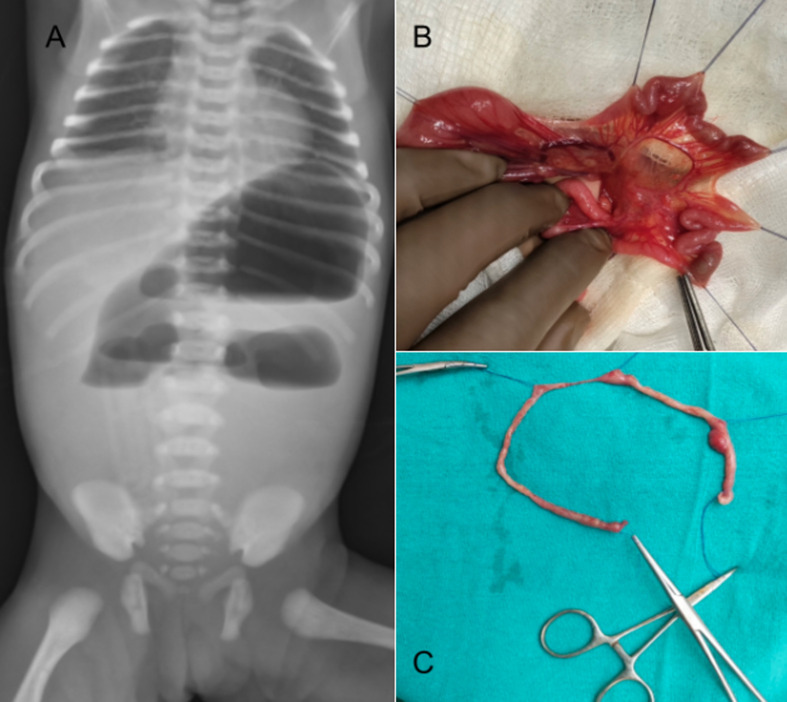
A) chest X-ray of the neonate showing a classical triple bubble sign; B,C) intraoperative photos showing multiple atretic segments of the jejunum
